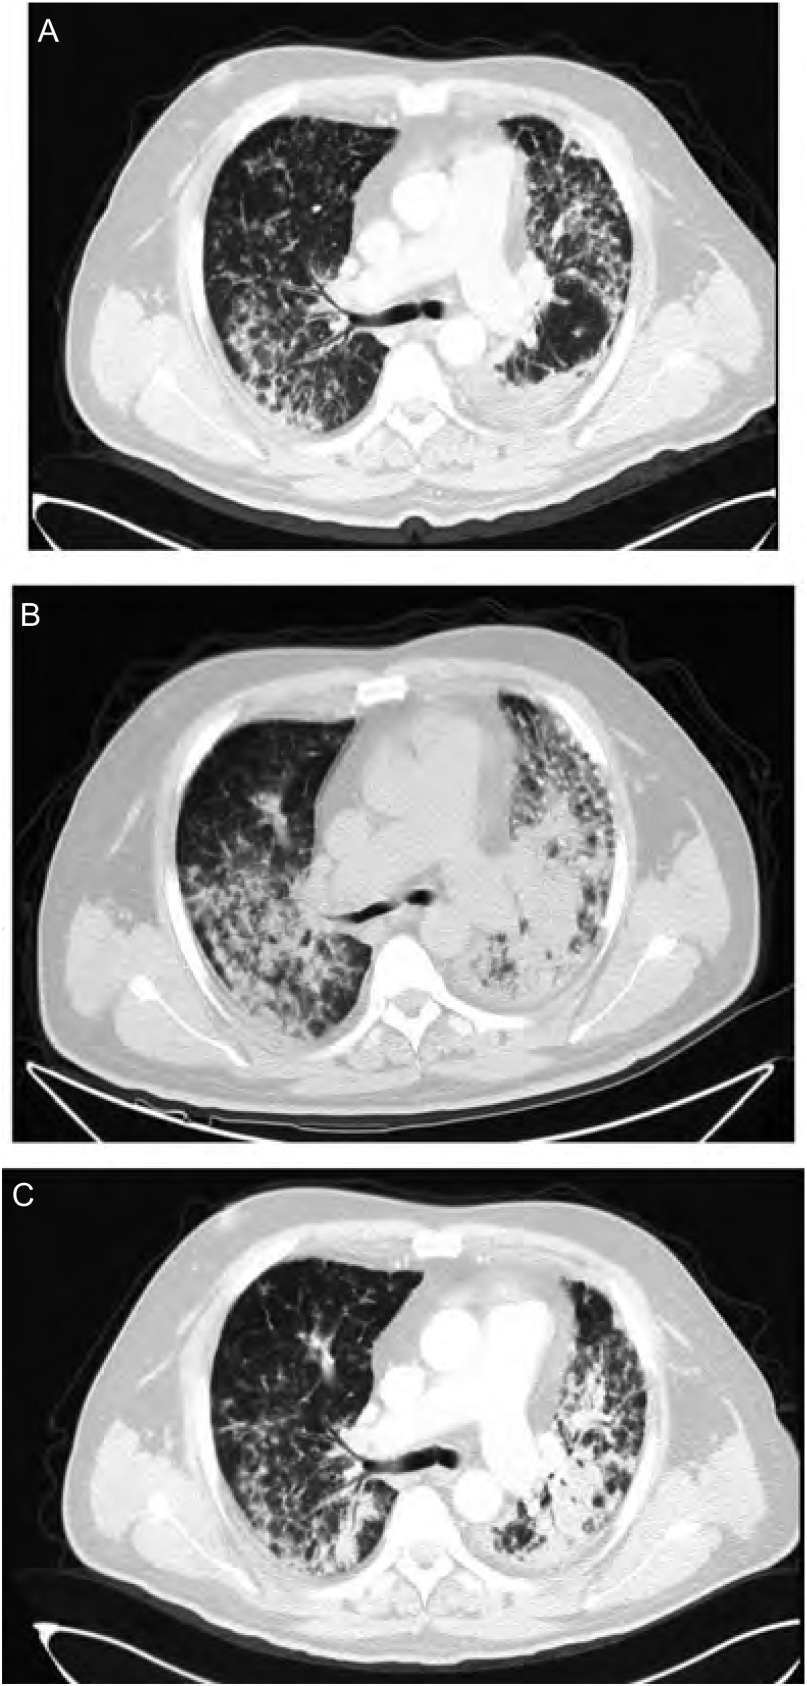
病例3厄洛替尼治疗前后及糖皮质激素治疗后胸部CT变化 Chest CT scans before and after erlotinib treatment and after corticosteroid treatment. A: Chest CT scan before erlotinib treatment; B: Chest CT scan after 21 days of erlotinib treatment; C: Chest CT scan after 21 days of corticosteroid treatment.
